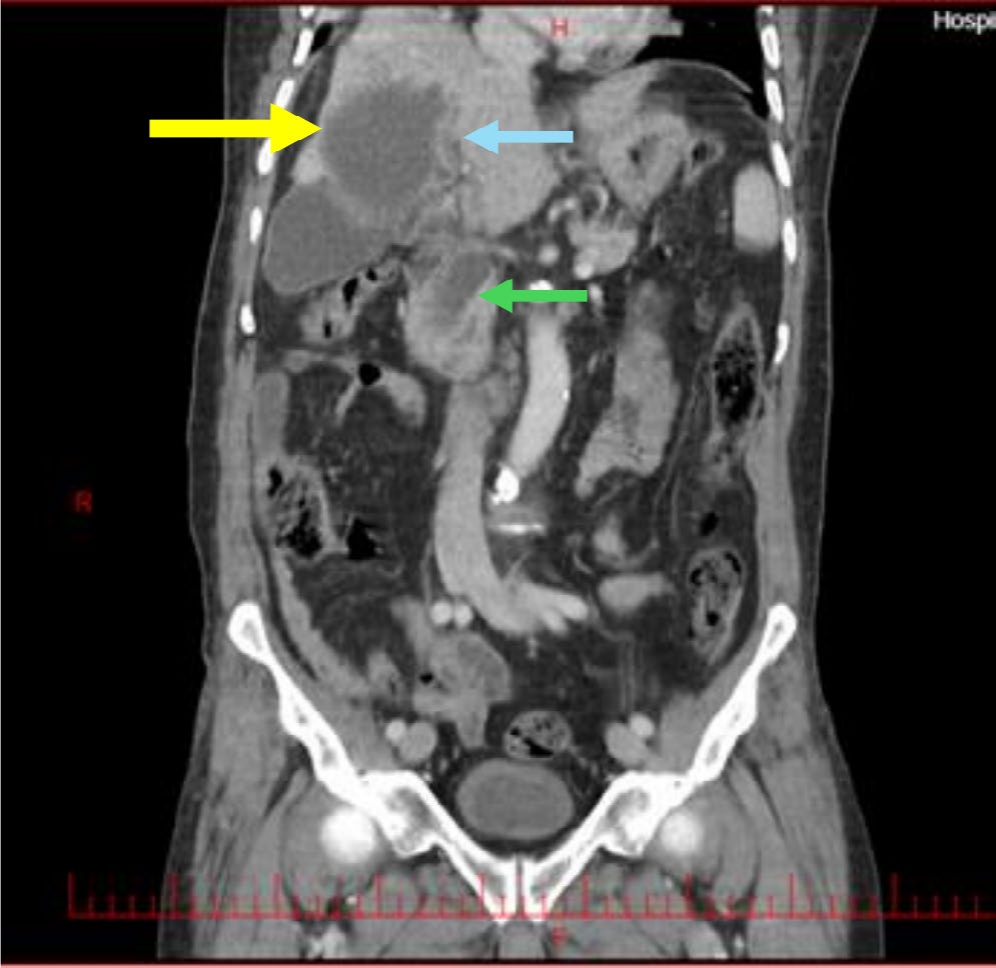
CT of the abdomen (coronal view). A hydatid cyst is observed in the right lobe of the liver (yellow arrow), alongside a fistula connecting the cyst to a branch of central biliary tree (blue arrow), which leads to the CBD (green arrow).
